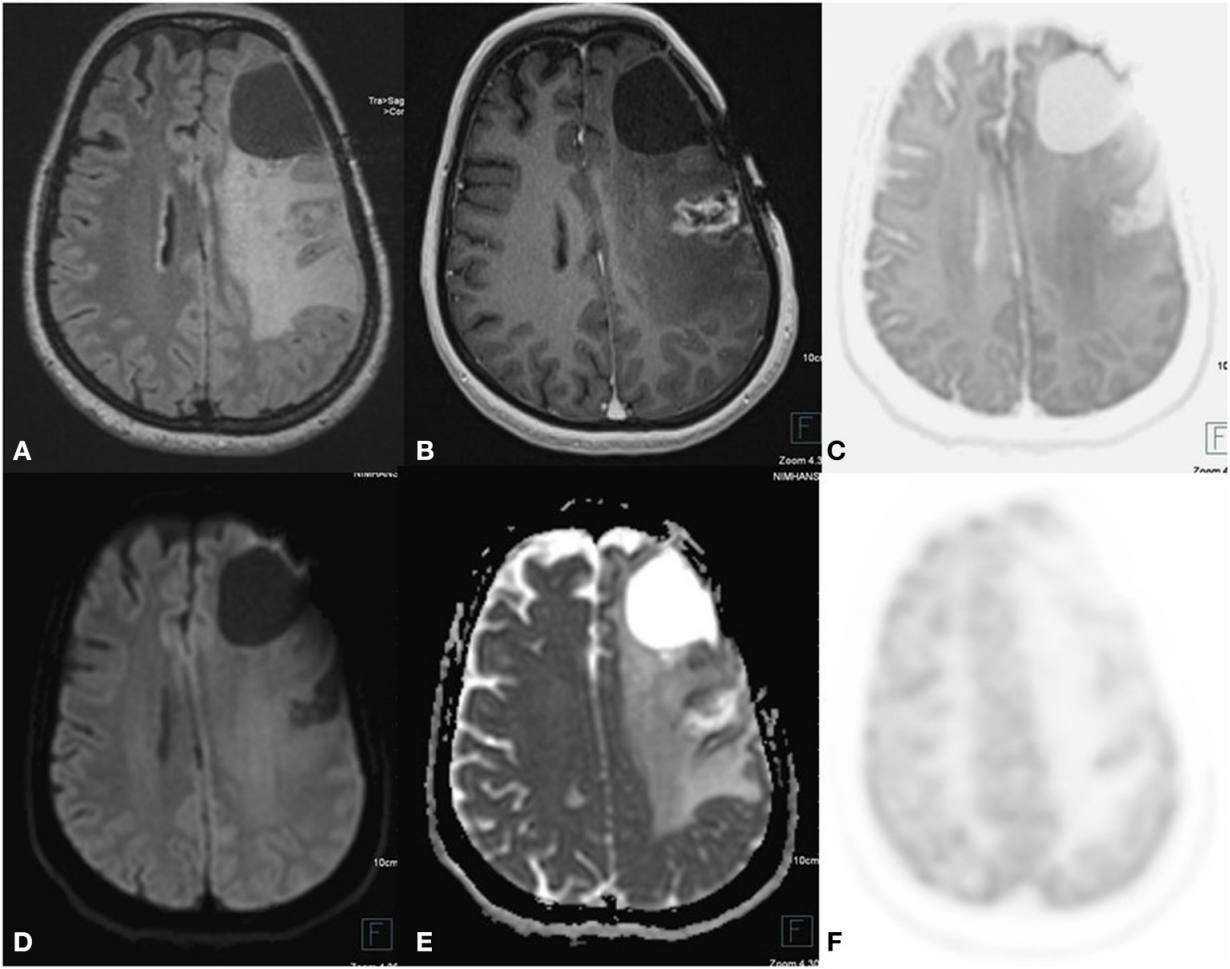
A 24 year old female postoperative, post-RT case of Glioma left frontal lobe, 3 year follow up MRI FDG PET axial images T2-FLAIR (A) post-contrast T1 (B) Inverted DWI (C) DWI b2000 (D) ADC (E) NAC PET (F) show a postoperative cavity in the left frontal lobe with Swiss cheese-like enhancing lesion posterior to postoperative either no elevated perfusion. Neither DWI restriction nor elevated metabolism on PET is seen, which is suggestive of radiation necrosis.
